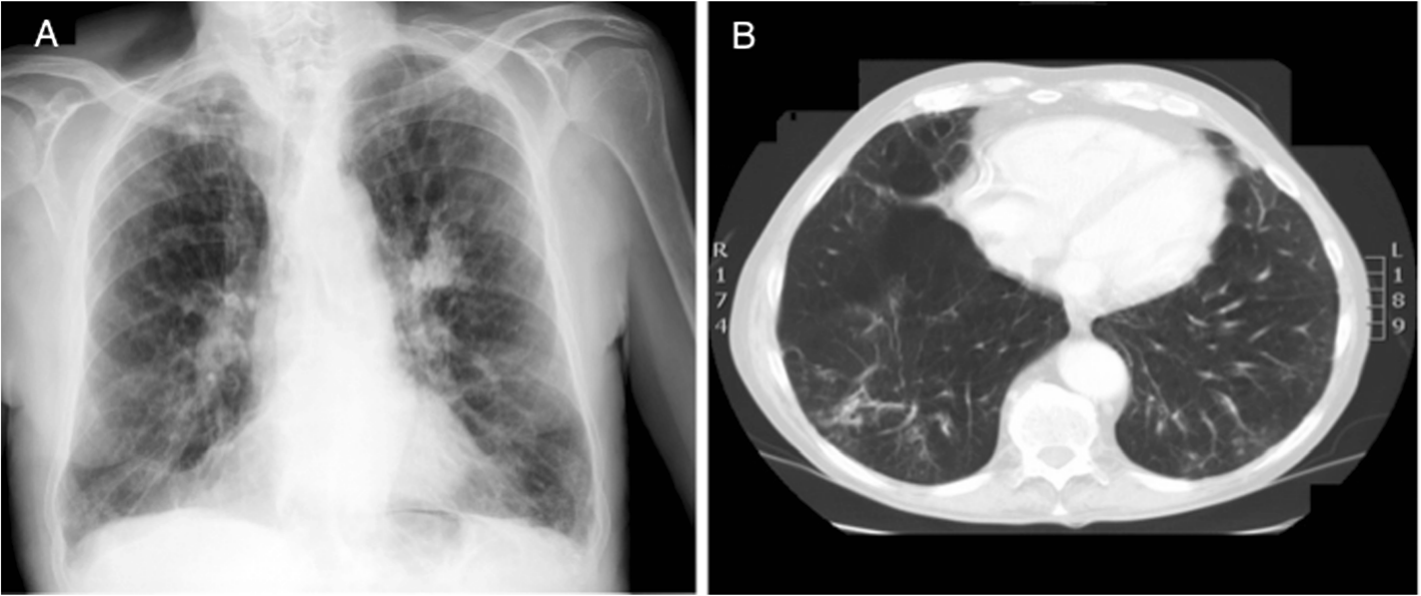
a Posteroanterior chest x-ray showing almost complete resolution of the right lower lobe alveolar infiltrate. b Chest computed tomographic scan showing almost complete resolution of the right lower lobe consolidation.
